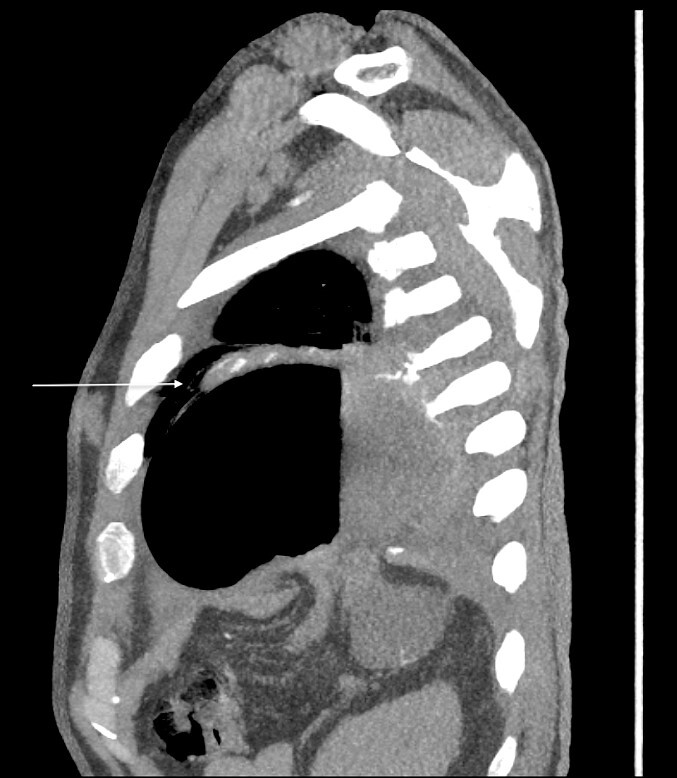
Sagittal CT showing gastric body herniating through diaphragmatic defect and the “dangling diaphragm” sign (arrow).
